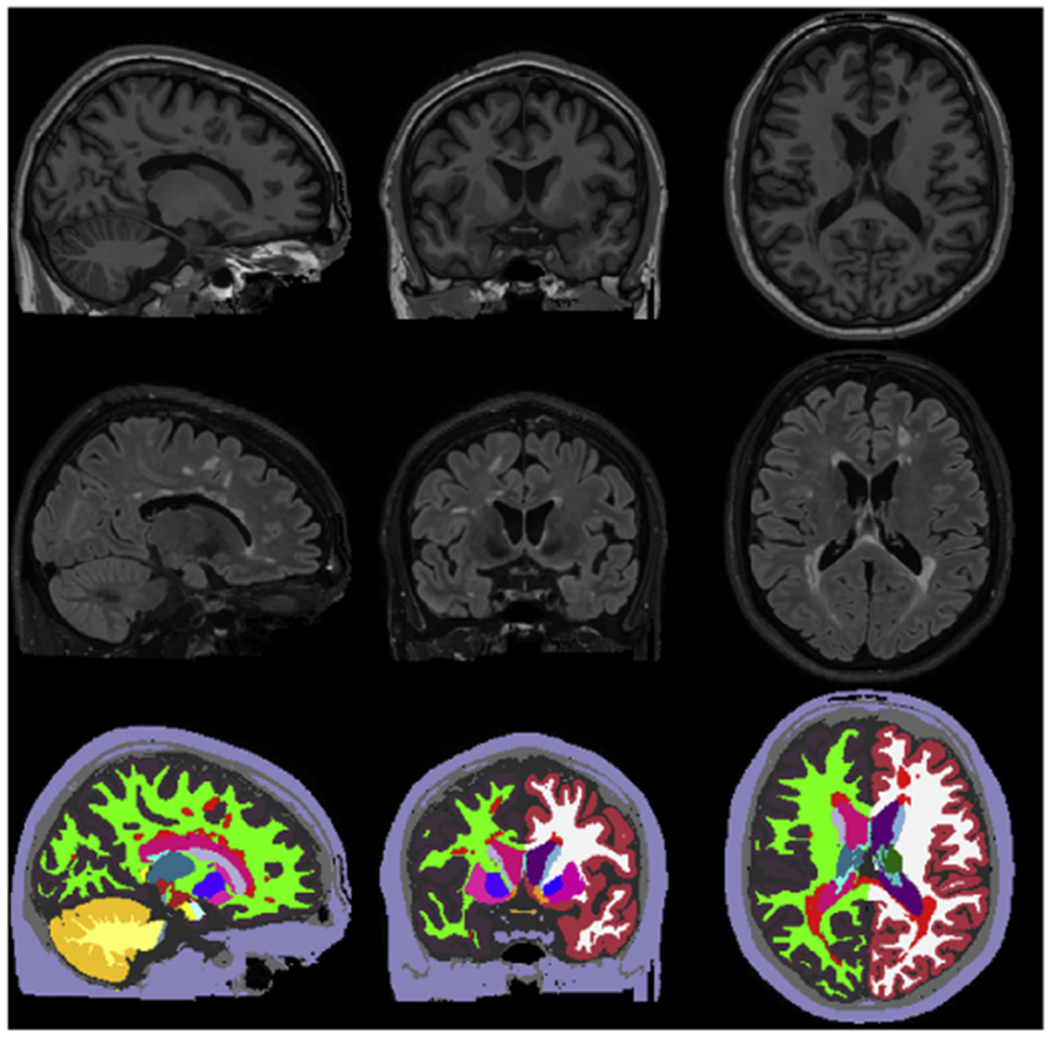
Segmentation of white matter lesions and 41 different brain structures from the proposed method on T1w-FLAIR input. From left to right: sagittal, coronal, axial view. From top to bottom: T1w, FLAIR, automatic segmentation.
